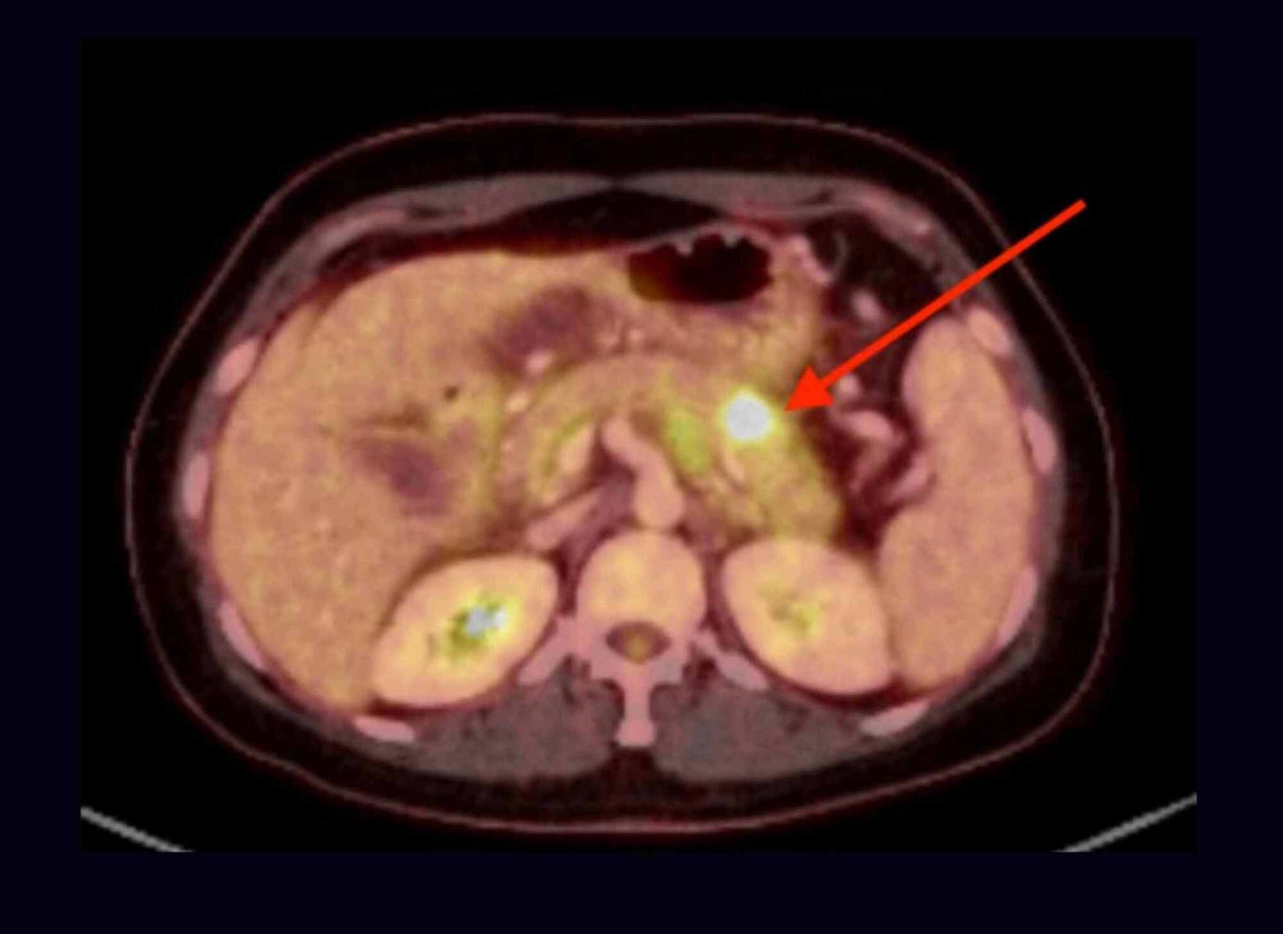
PET/CT showing focal uptake of FDG by a 1.8 cm round and hypodense lesion (red arrow) in the body-tail of the pancreas (max SUV of 11) PET/CT: positive emission tomography/computed tomography; FDG: fluorodeoxyglucose; cm: centimeter; SUV: standardized uptake value
